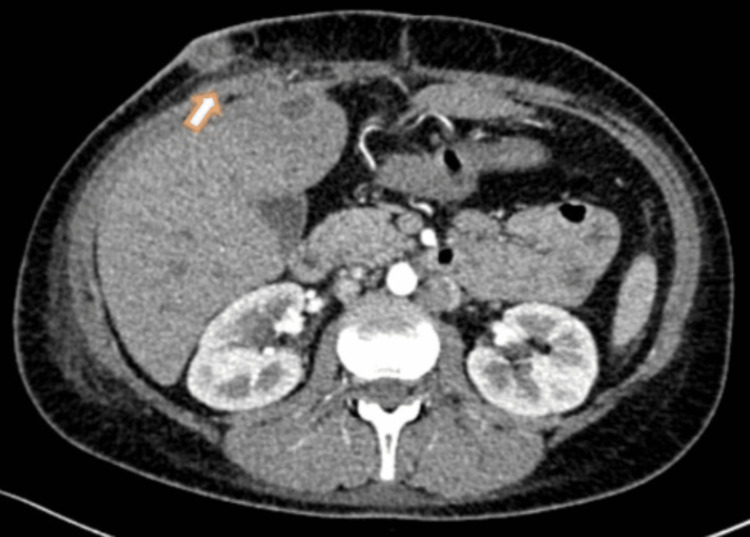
Abdominal CT scan shows an abscess anterior to segment IV of the liver, connected from one side with the ileocolic anastomosis and from the other side with a fistula tract (white arrow) that opens in turn in the anterior abdominal wall.
